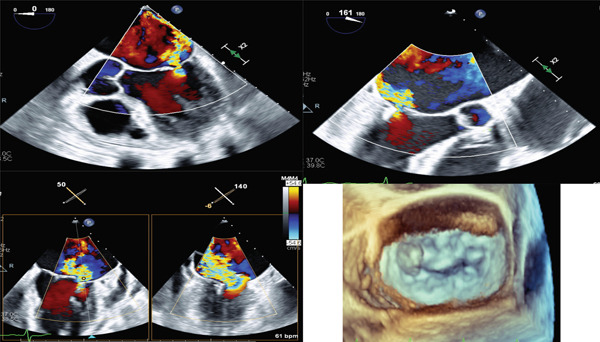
A broad jet with significant suction flow from the AC/A1 region reached the base of the left atrium. A straight jet with broad suction flow was also observed from the A2/P2 region. Detachment of the clip at the AC region was noted, along with prolapse of both the AC and A1 segments. Functional MR was present from A2/P2. Both the AML and PML showed evidence of tethering. TEER = transcatheter edge‐to‐edge repair, TEE = transesophageal echocardiography, AML = anterior mitral leaflet, PML = posterior mitral leaflet, and MR = mitral valve regurgitation.
